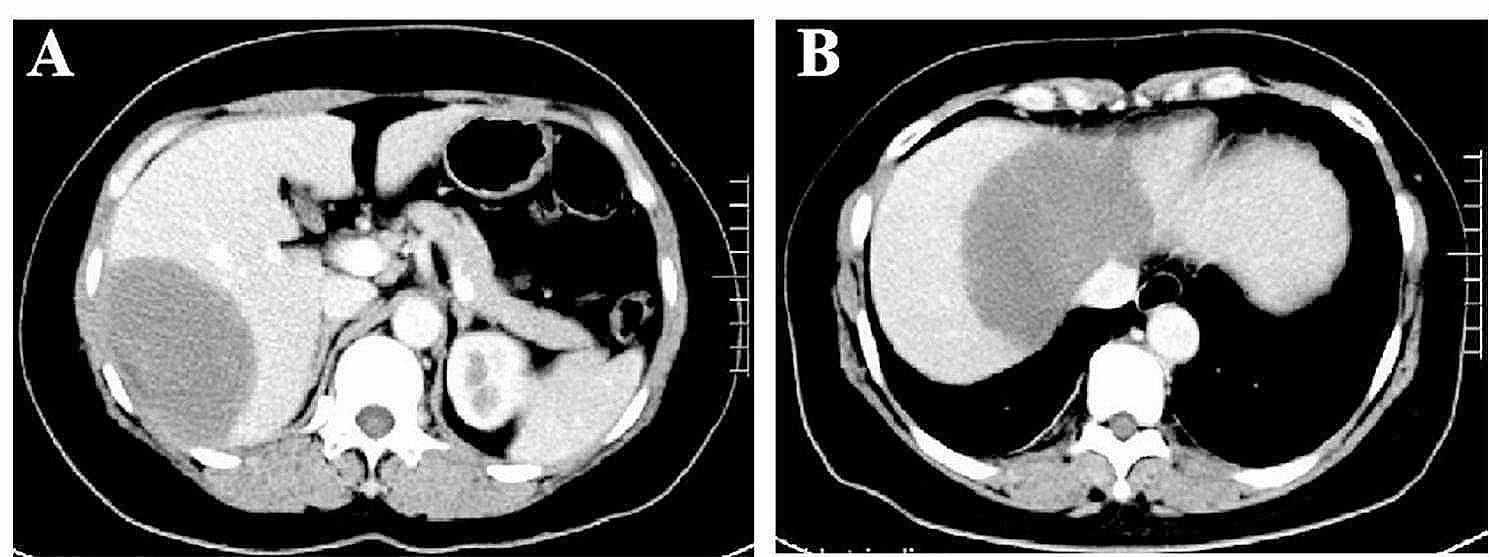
Abdominal enhanced computed tomography of the patient. The abdominal computed tomography performed on 5 days prior to admission, shows one space-occupying lesion each in the right lobe (6.4 × 9.0 × 5.0 cm) (A) and left lobe (B)
